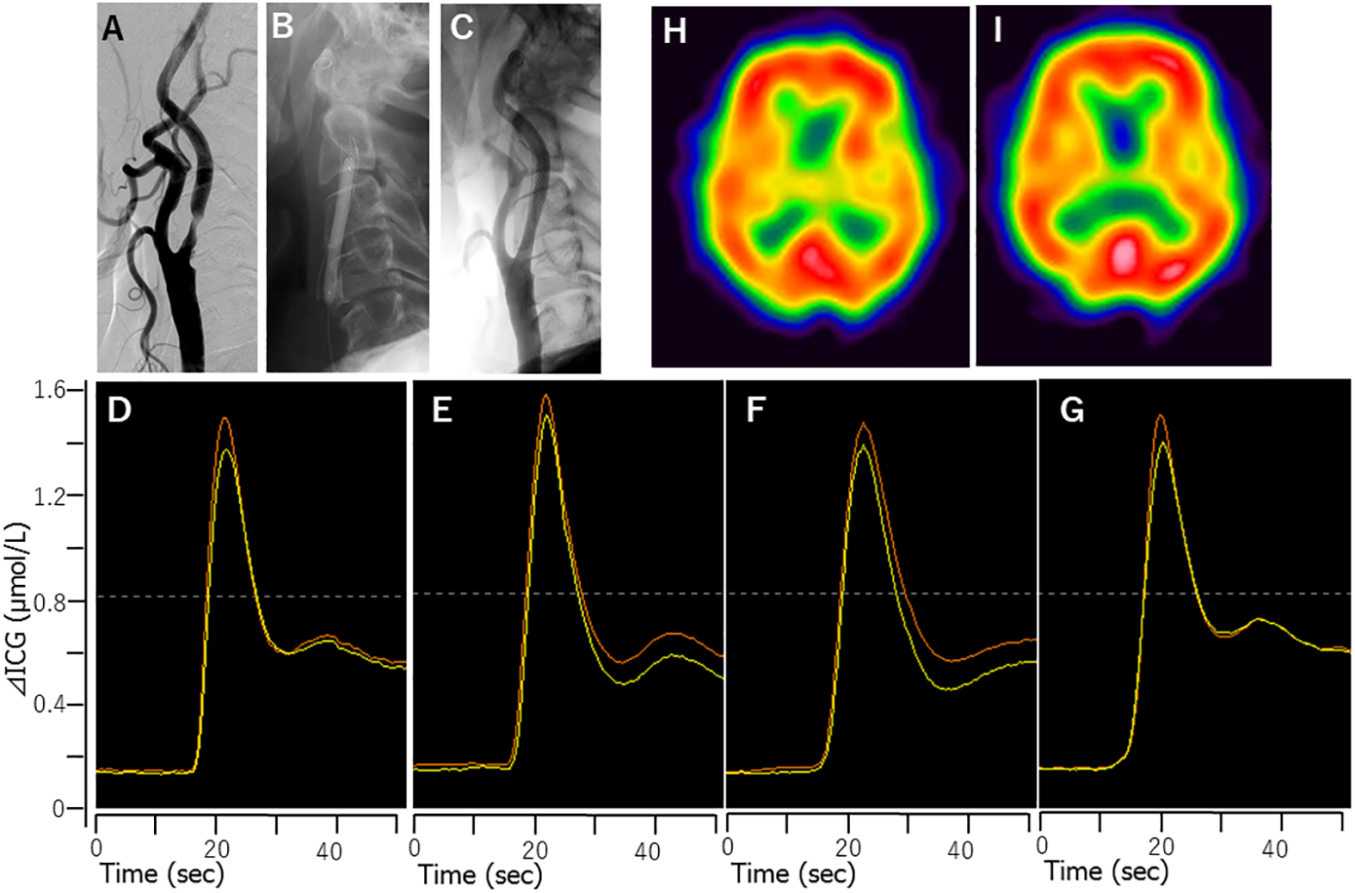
Case 1 (non-HP): A 64-year-old male presented with severe symptomatic right internal carotid artery stenosis. Right common carotid angiography showed severe right internal carotid artery stenosis (A). Balloon angioplasty for post-dilatation was performed after stent placement with filter protection (B). Right common carotid angiography after CAS confirmed stent patency (C). Time-intensity curve from ICG-NIRS monitoring before (D), just after (E), 6 hours after (F), and 1 day after CAS (G). No remarkable change was seen in the time-intensity curve time course throughout the measurement. Yellow curve; affected side, Orange curve; unaffected side. We found no remarkable differences between preoperative rCBF in the right hemisphere with ^123^I-IMP SPECT in the resting state between before (H) and 1 day after CAS (I). MRI showed no additional ischemic lesions. He was discharged without any neurological deficits.
